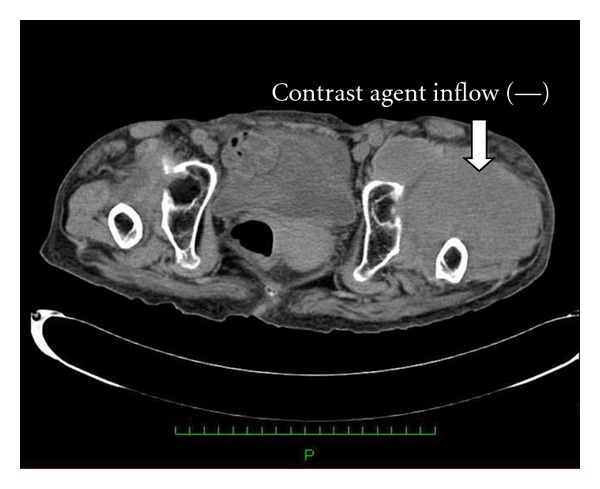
Contrast-enhanced CT scan at 2 months after the embolization.
